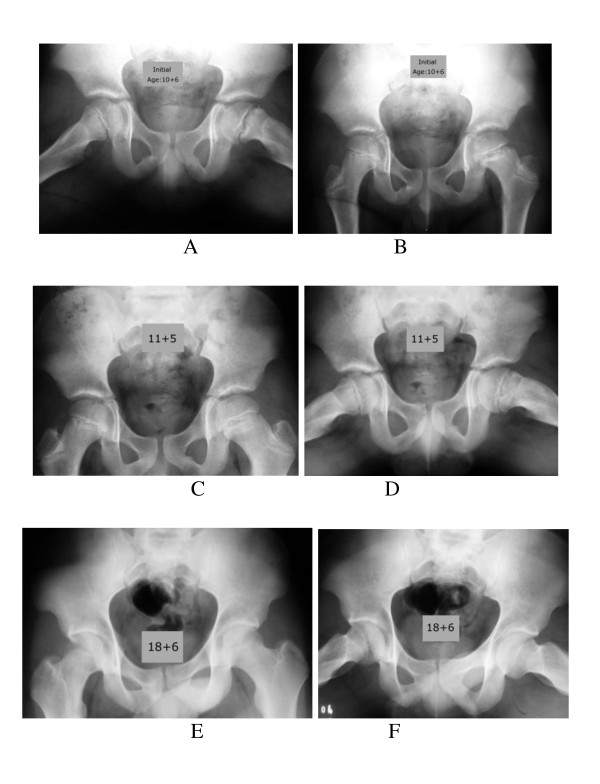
Early slipping of the femoral epiphysis of the left hip. A cast after twelve weeks was applied. The image of the left hip shows growth arrest andno progression with conservative management. (A and B) Anteroposterior and frog-leg lateral radiographs of the pelvis made before treatment, showing the zone of rarefaction on the metaphyseal side in the left hip of the growth plate in Chronic/Mild SCFE, in a ten and half year old boy. (C and D) Anteroposterior and frog-leg lateral radiographs eight months after spica cast had been discontinued. The rarefaction zone has diminished and persists in the left hip. (E and F) Final result. The growth-plate has completely closed on both radiographs of the left hip.
